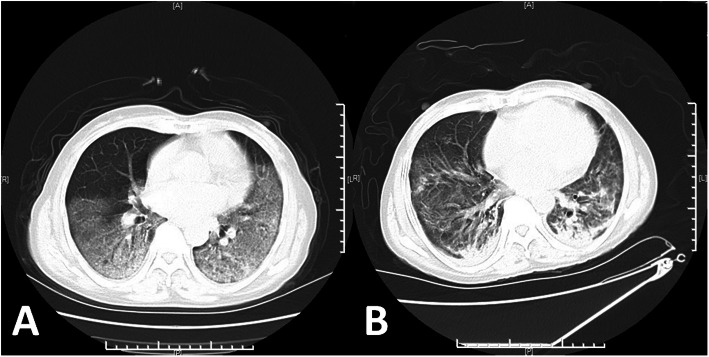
a Chest CT on the 8th day of admission. b Chest CT on the 39th day of admission
